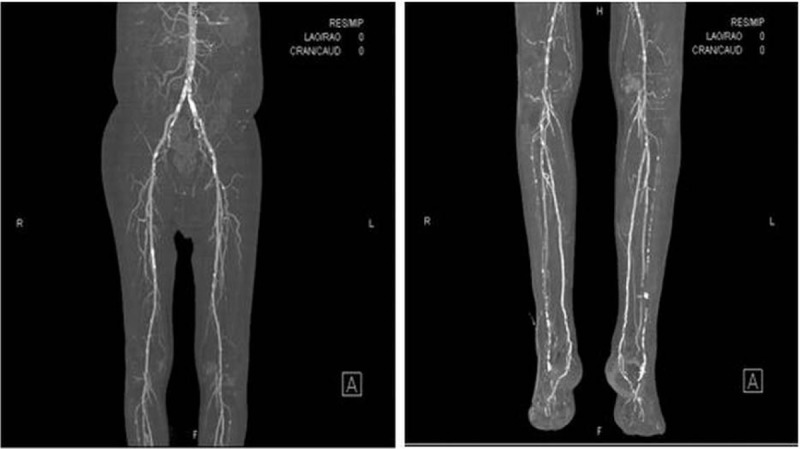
Computed tomography (CT) angiography of diabetic foot. (Left) CT angiography of the thigh. (Right) CT angiography of the lower leg.
